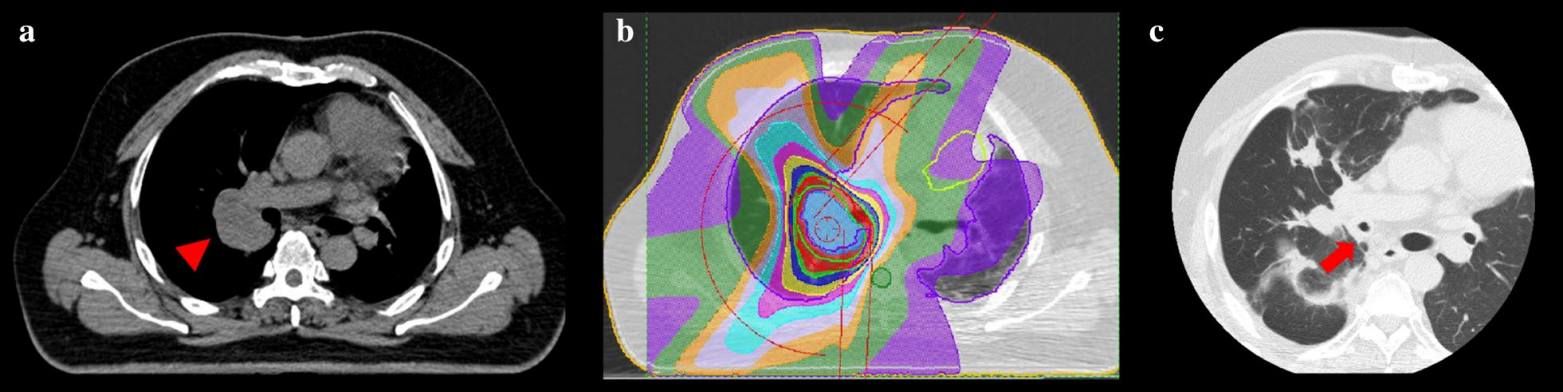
Computed tomography images before and after radiation therapy. a A metastatic tumor in the right hilum (red arrowhead). b Treatment plans for stereotactic body-radiation therapy. c Axial view after radiation therapy showing stenosis of the right main bronchus (red arrow)
